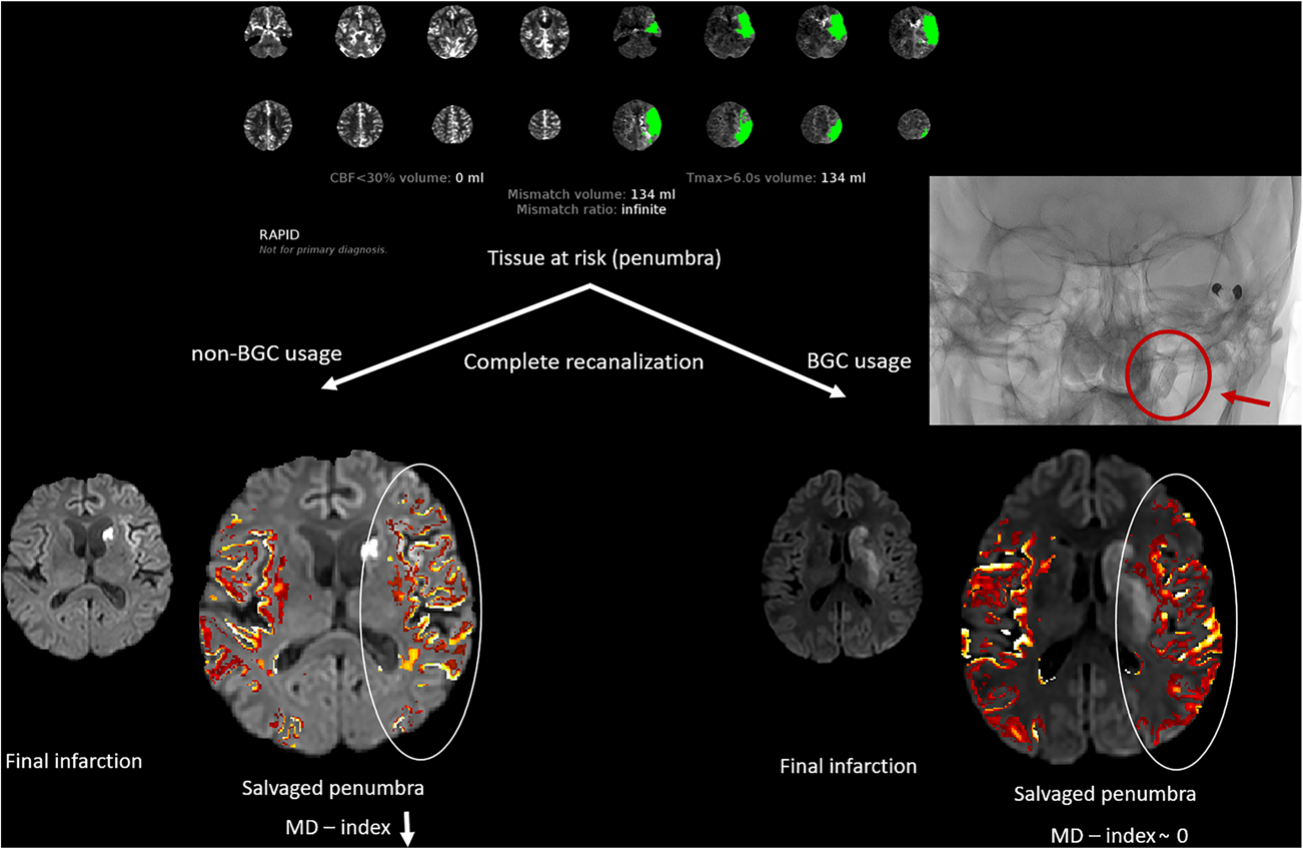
Examples of assessing microstructural integrity of the salvaged penumbra (representative example of RAPID evaluation in CT perfusion imaging). After complete recanalization of an acute occlusion of the middle cerebral artery, MD values of the affected side are lowered in comparison to the contralateral side only for the case of non-BGC usage. BGC, balloon guide catheter
